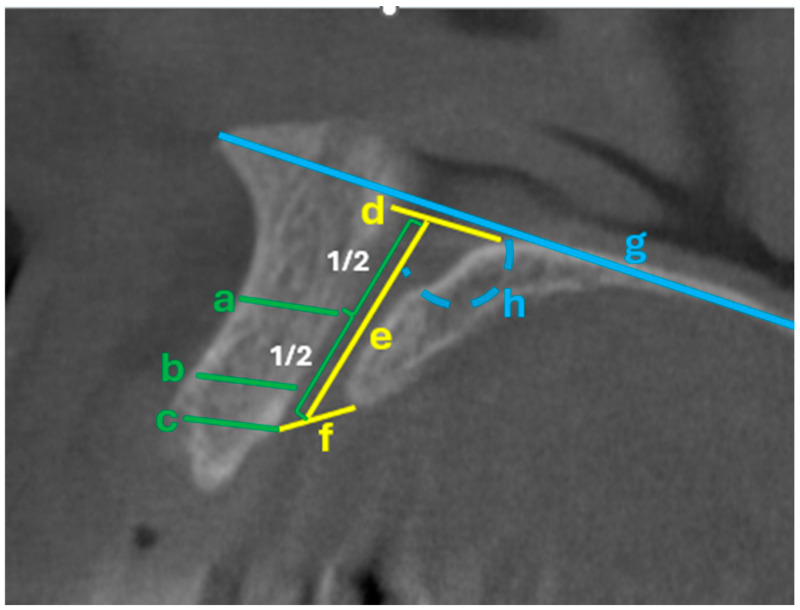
The following landmarks were selected for analysis of the sagittal CBCT images [all measurements in mm (except h; h shows the angle in degrees)]; (a) cranial reading evaluates the distance from the buccal border in the middle of the nasopalatine canal to the facial aspect of the buccal bone wall. (b) Distance midway from the buccal bone wall of the nasopalatine canal to the facial aspect of the bone wall using a horizontal line from the palatal border of the incisive foramen. (c) Crestal distance from the buccal border of the incisive foramen to the facial aspect of the buccal bone plate. (d) The diameter of the nasopalatine foramen. (e) The length of the nasopalatine canal. (f) The diameter of the incisive foramen. (g) Palatal plane. (h) Angle formed by the nasopalatine canal and the palatal plane.
